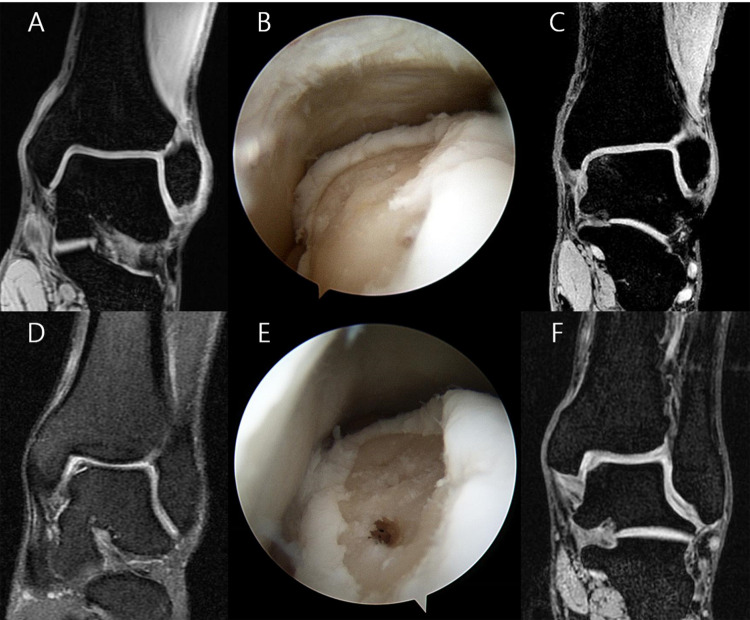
(A) In a 53-year-old male smoker, a preoperative coronal T2-weighted MRI shows an osteochondral lesion of the talus with fibrillated cartilage and no evidence of subchondral bone edema. (B) An arthroscopic image taken after microfracture treatment corresponds to Ferkel and Cheng stage D. (C) A follow-up T2-weighted MRI performed 3 years postoperatively reveals a minor hypointense signal and split-like defect at the repaired cartilage with mild subchondral edema (MOCART score 70 points). (D) In a 21-year-old male non-smoker, a preoperative coronal T2-weighted MRI reveals an osteochondral lesion on the talus with a fully detached but non-displaced fragment. (E) An arthroscopic image taken following microfracture treatment, classified as Ferkel and Cheng stage E. (F) A follow-up T2-weighted MRI performed 2.7 years postoperatively shows the defect completely filled with repair tissue and full integration with adjacent cartilage without subchondral change (MOCART score 85 points).
